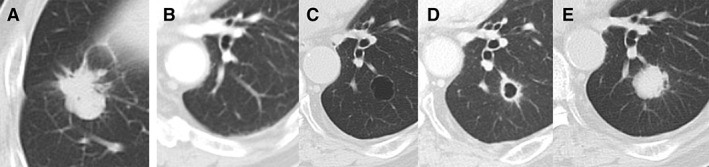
Chest computed tomography (CT) findings. (A) An irregular nodular opacity is detected in the right lower lobe, which was diagnosed as a primary lung cancer. (B) No abnormal finding in the left lower lobe is seen on the chest CT performed one year before the CT shown in (A). (C) At the same time of the CT examination of (A), a thin‐walled cyst was detected in the left lower lobe. (D) The cyst wall became thicker as seen in the image obtained one year after the lung cancer surgery. (E) In the CT obtained five months after the CT shown in (D), a nodular shadow is identified in the left lower lobe at the same site as the cavity lesion.
